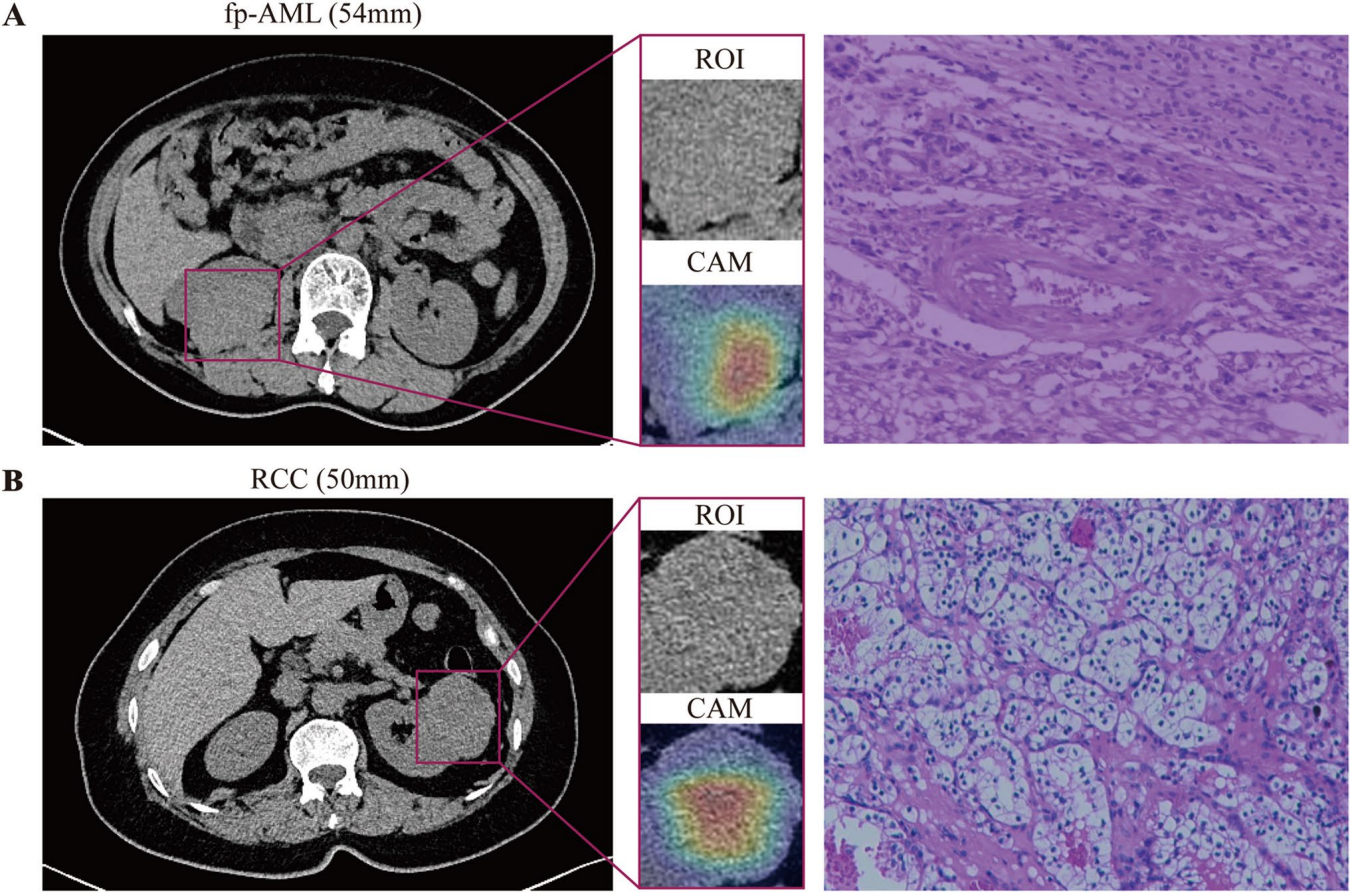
Representative example predictions from Sun Yat-Sen University Cancer Center (SYSUCC). a An unenhanced CT image from a 50-year-old woman who was preoperatively diagnosed with renal carcinoma but eventually confirmed by pathology as fat-poor angiomyolipoma (fp-AML). Due to a misdiagnosis of the tumor, she underwent a radical nephrectomy and lost the chance to preserve her right kidney. Our model successfully identified this tumor as fp-AML. b An unenhanced CT image from a 54-year-old woman who underwent a partial nephrectomy and was pathologically confirmed as clear cell renal cell carcinoma (ccRCC). Our model successfully identified this tumor as renal cell carcinoma (RCC). *ROI* region of interest, *CAM* class activation mapping
